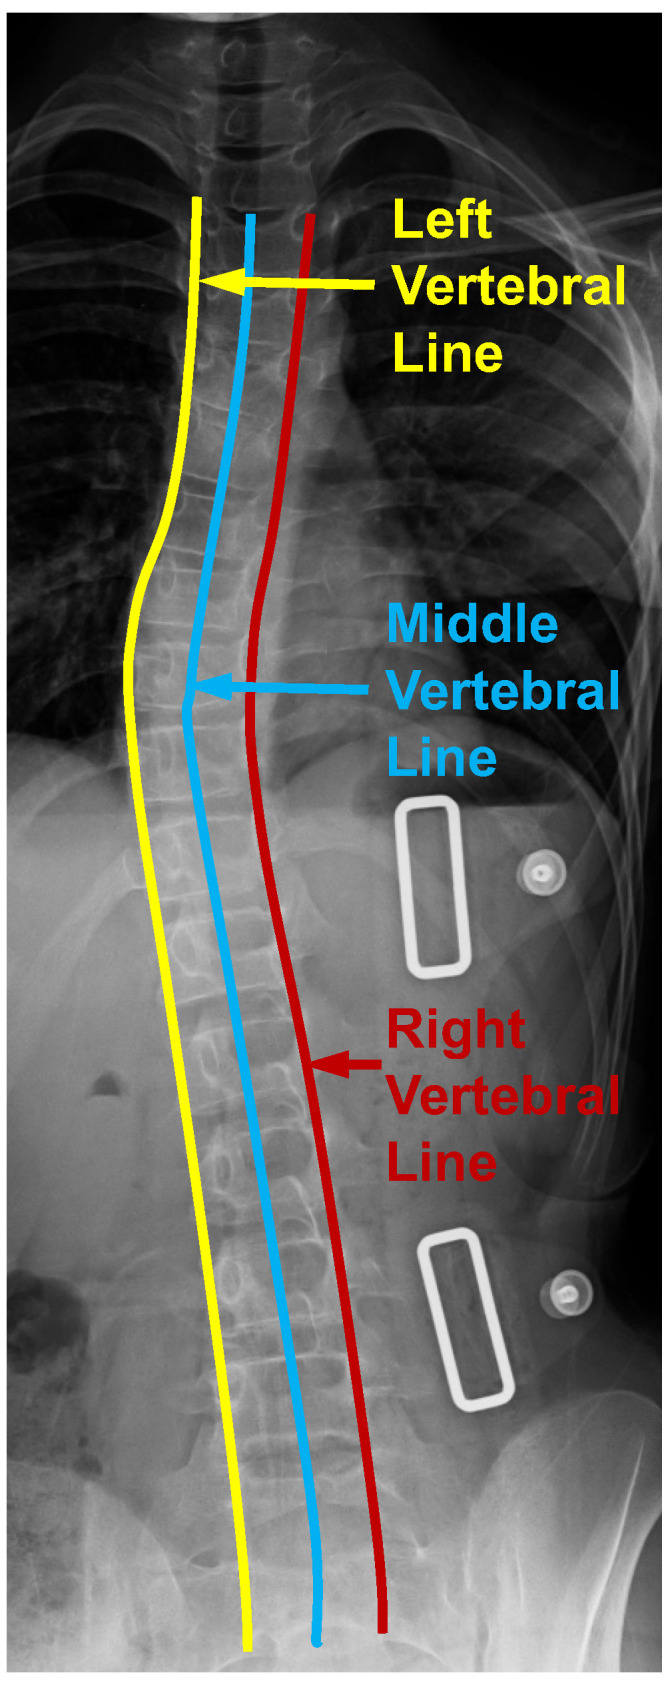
Three vertebral lines on the spinal anterior–posterior X-ray images, including the left, middle, and right vertebral lines.
